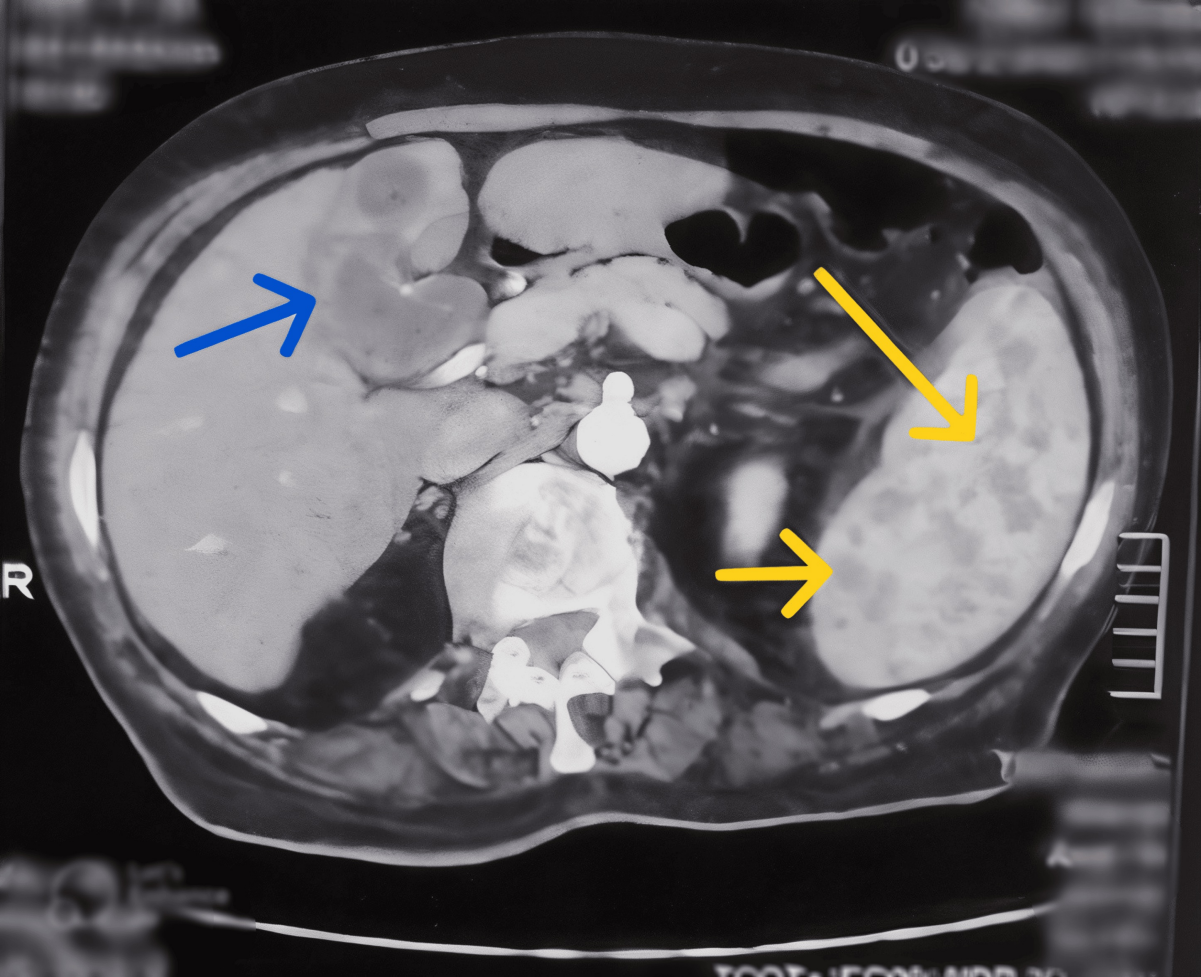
CT scan of the abdomen showing hypoechoic foci in the liver (blue arrow) and spleen (yellow arrows)
